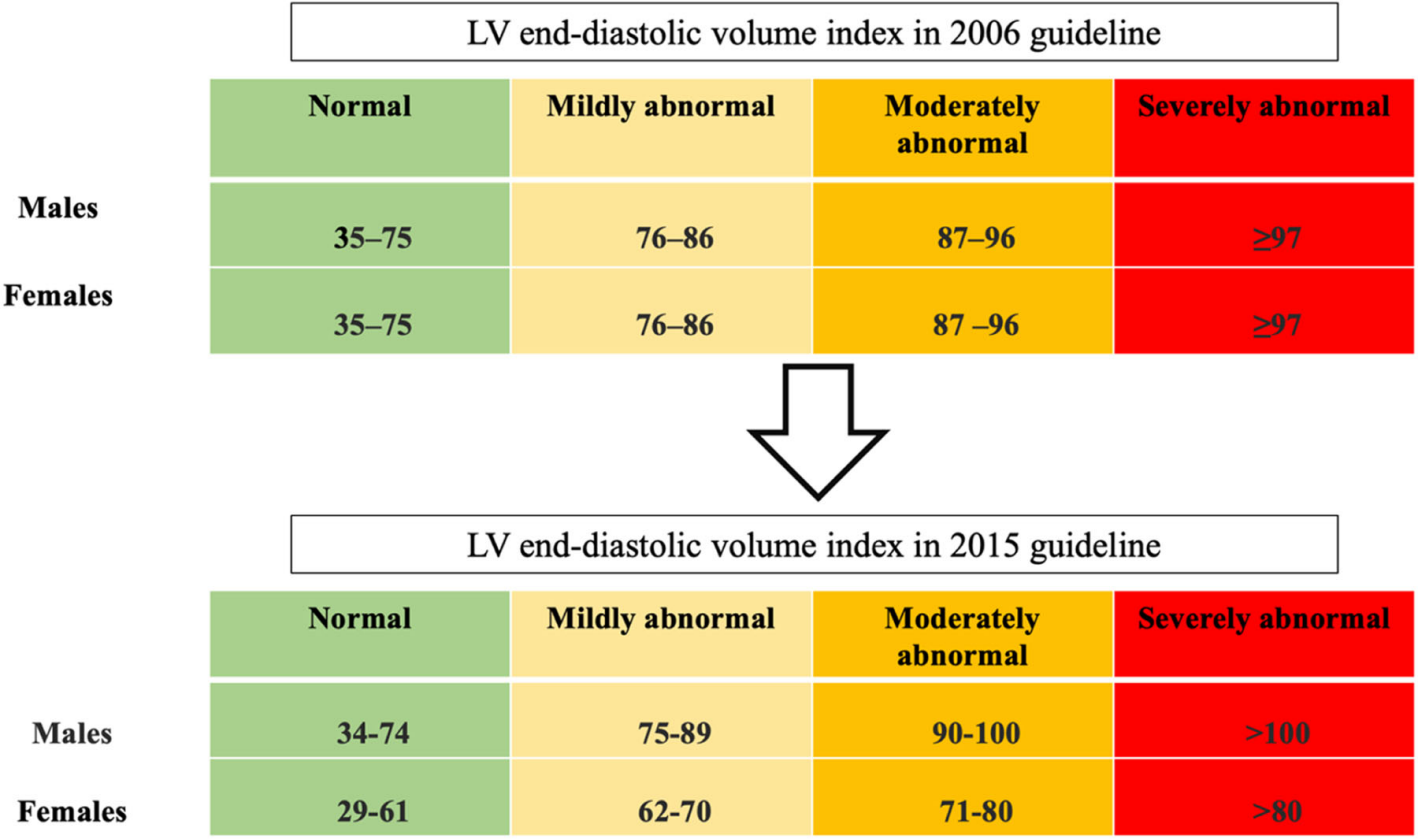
Reference ranges and partition cutoff values for 2D echocardiography‐derived LV end‐diastolic volume index in 2006 and 2015 guidelines in men and women.
